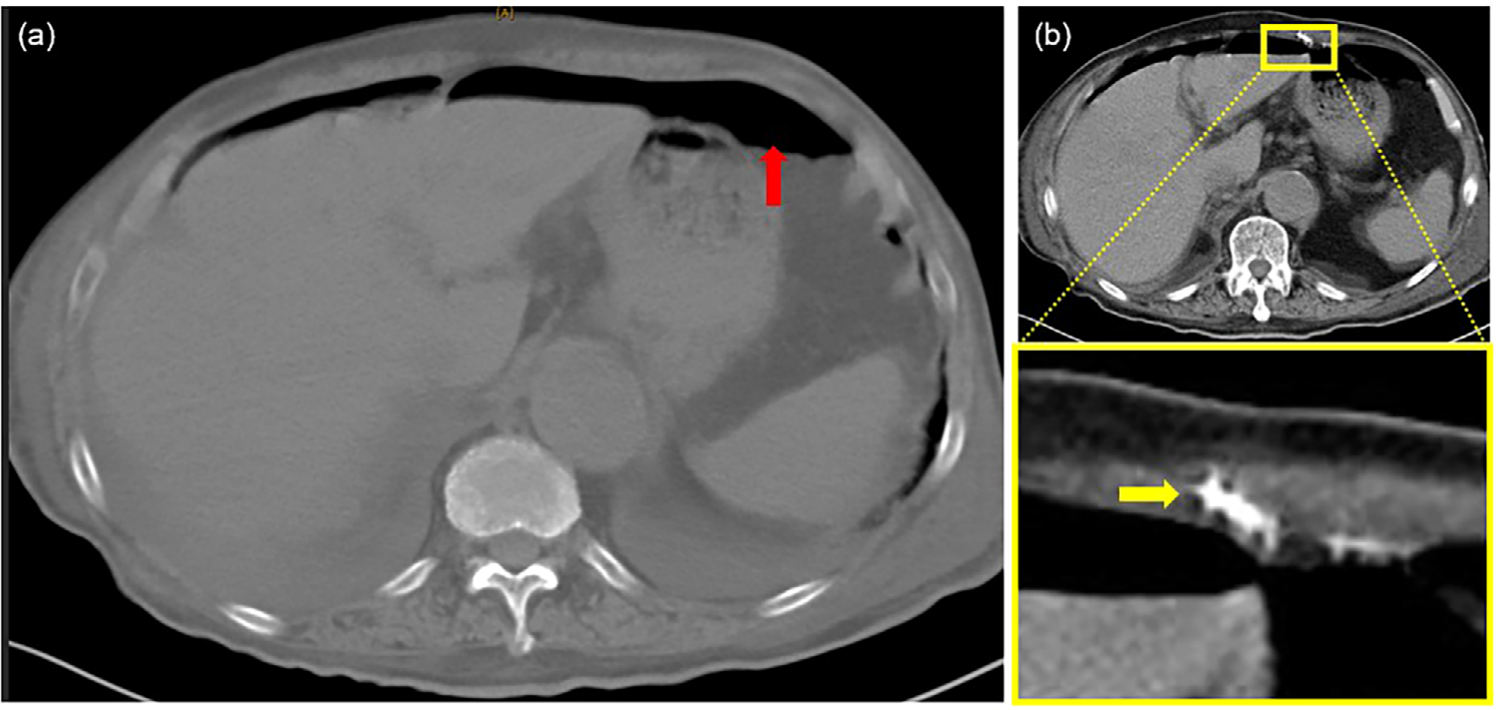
Abdominal computed tomography (CT) on day 6 After gastrostomy placement (a) A large amount of free air is observed (red arrow). (b) The gastric wall fixation device has migrated into the abdominal wall (yellow arrow).
